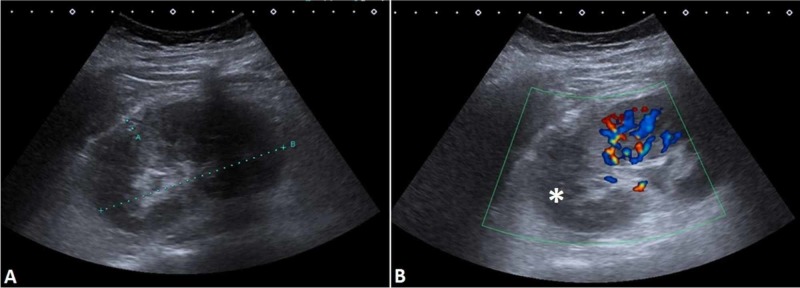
(A) B-mode US of the affected kidney. (B) Color Doppler demonstrated a lack of blood flow in the upper pole of the kidney (asterisk). US, ultrasound
